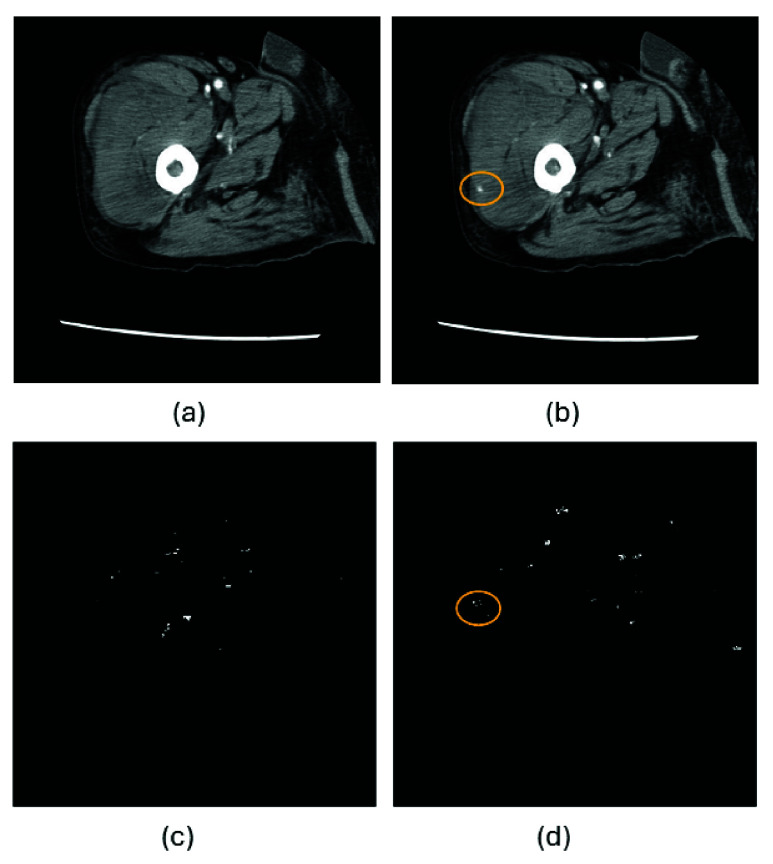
Sequential CT slices of thigh hematoma and corresponding KGA outputs for contrast extravasation detection. (a)-(b) Consecutive axial CT slices, with a surgeon-identified extravasation focus circled in (b). (c)–(d) Binary activation maps generated by the KGA, where white pixels indicate candidate extravasation regions and a positive alert is produced because the confidence score exceeds the predefined threshold. New activations in (d) correspond spatially to the suspected extravasation region.
